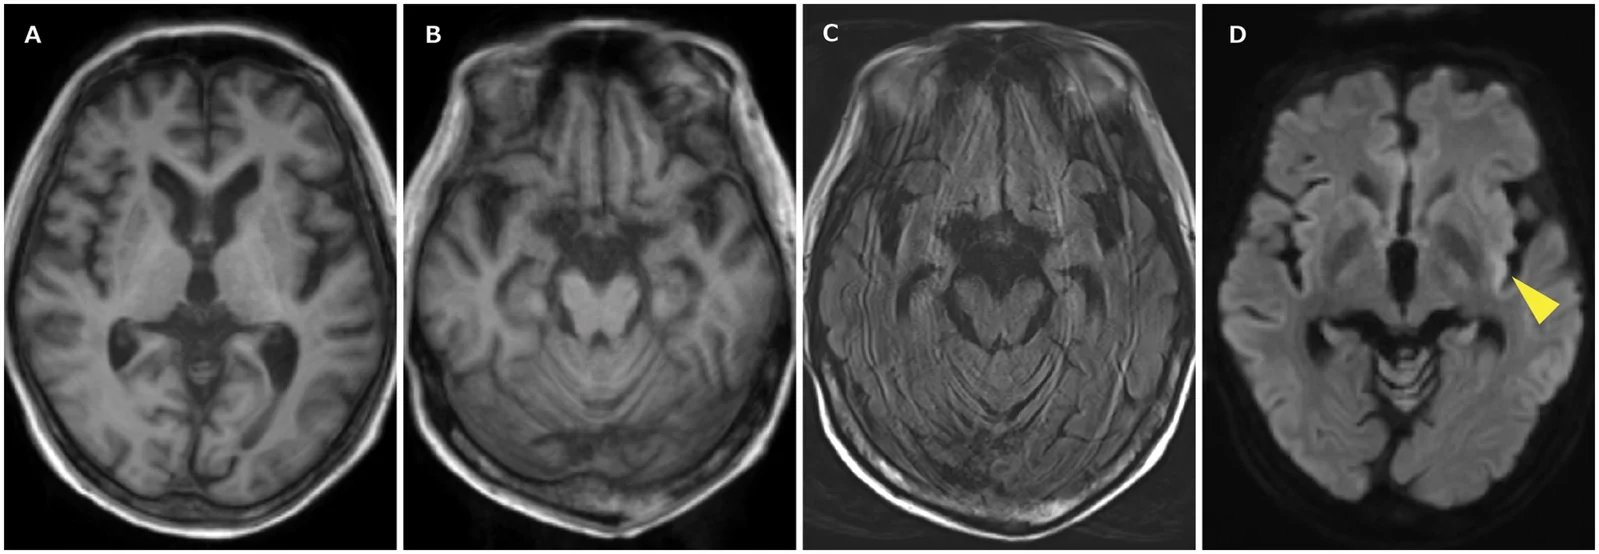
Brain MRI findings in a representative case of pellagra encephalopathy (Case 1). Brain MRI findings. Cerebral atrophy was not evident relative to the patient’s age on T1-weighted imaging (T1WI) (A), (B). A high-intensity area in the hippocampus was not evident on T2-FLAIR imaging (C). A mild high-intensity area was observed in the left caudal insula via diffusion-weighted imaging (DWI). This was considered a change due to an epileptic seizure (D).
